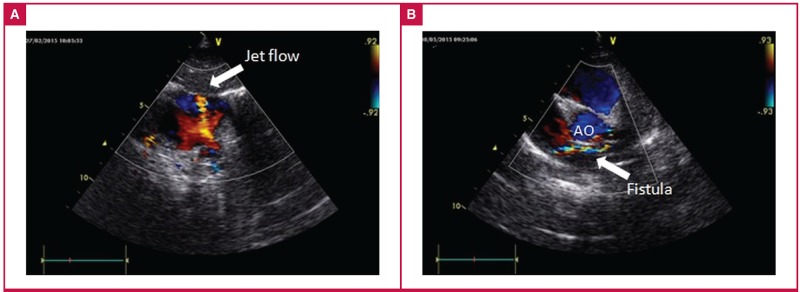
Pre-operative subcostal (A) and modified parasternal short-axis (B) echocardiographic views demonstrating the jet flow and trajectory of the coronary fistula.
